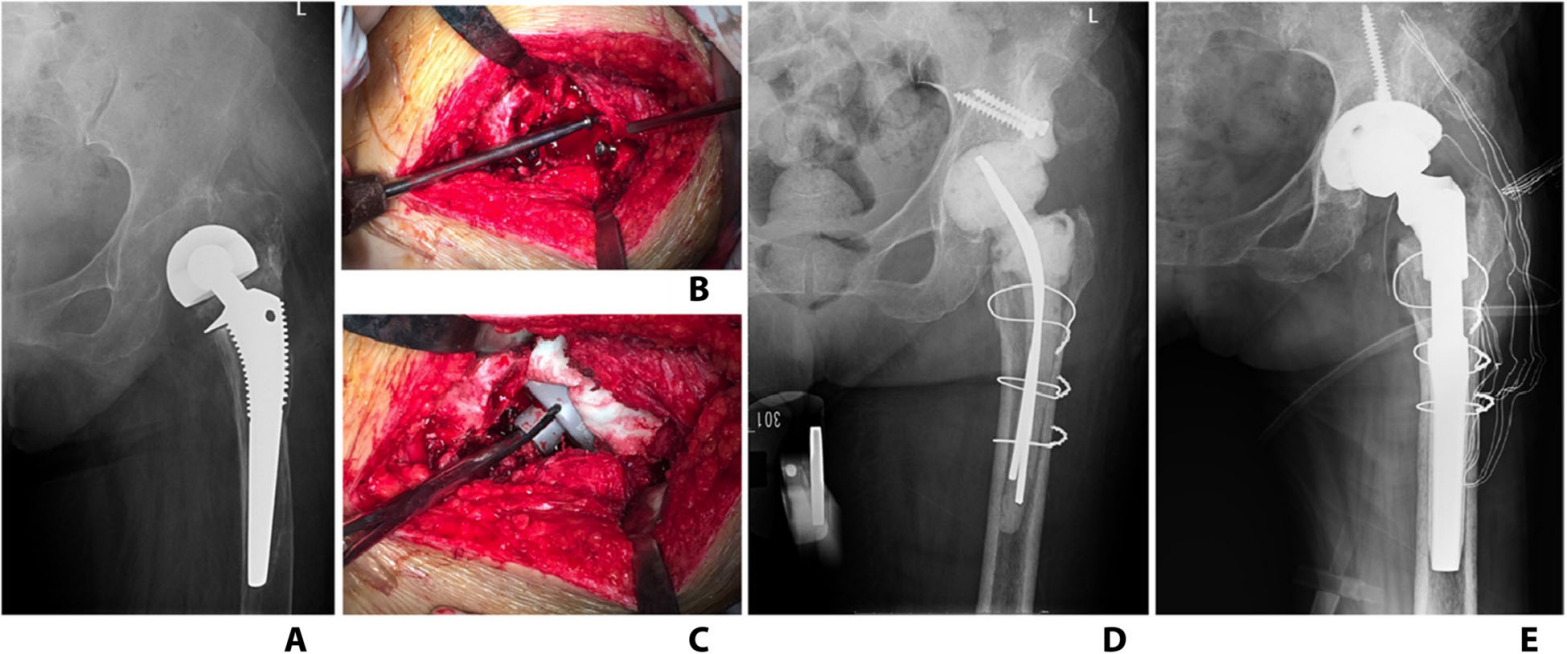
a One case with Paprosky IIB acetabular bone defect. b, c Screw-cement-shell was applied to reconstruct superior wall. d X-ray after spacer implantation. f X-ray after prosthesis implantation
